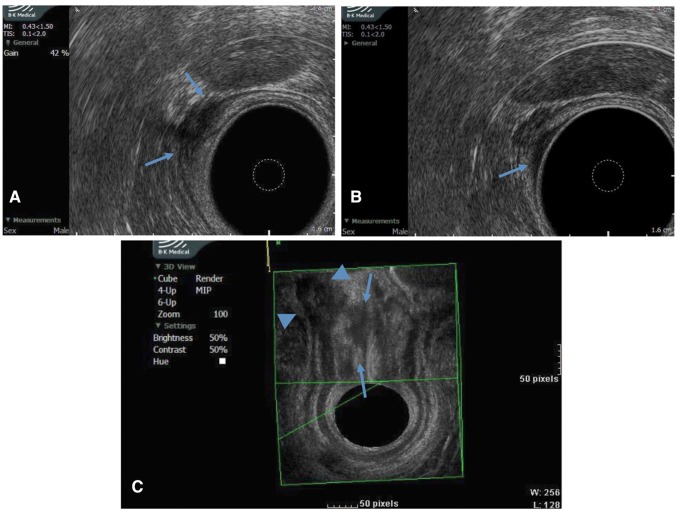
Endorectal ultrasound of rectal lesion showing submucosal involvement. (A) Axial view with arrows denoting mural component in muscularis propria; (B) Axial view with arrow demonstrating mucosal component; (C) 3D reconstruction showing mucosal (arrows) and muscular wall (arrowheads) components.
